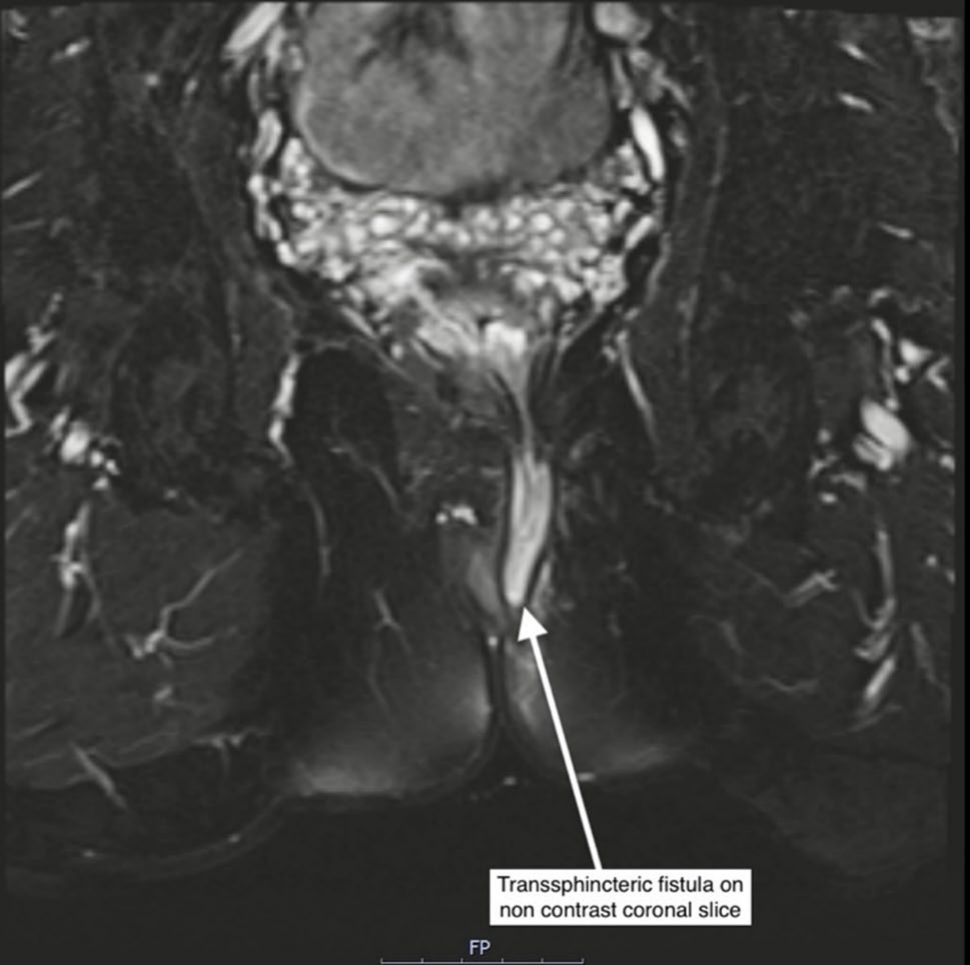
Transsphincteric fistula on noncontrast MRI coronal slice
